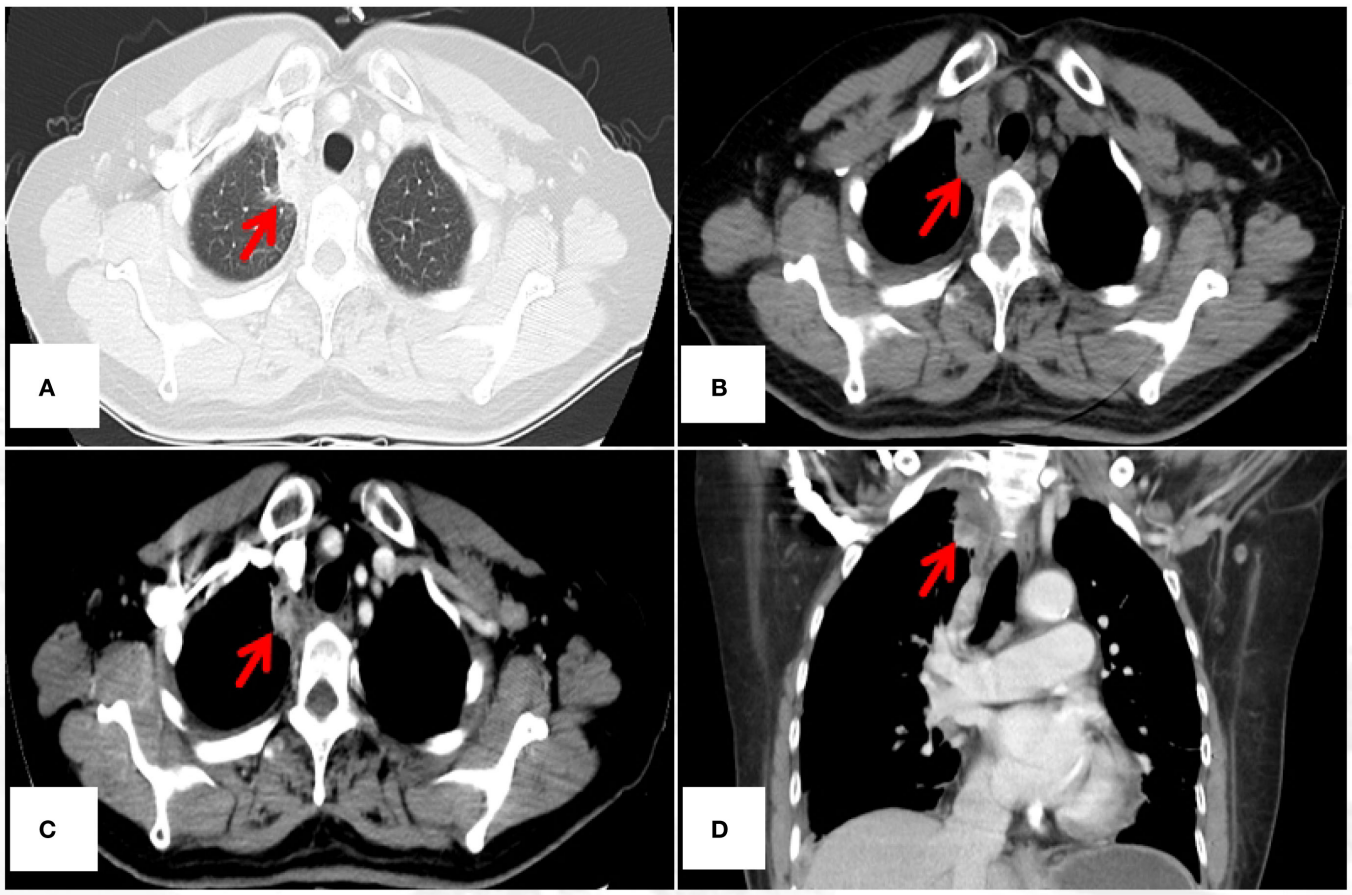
45-year-old female diagnosed with ETAC. Immunocytochemistry shows: Ber - EP4(+), CK7(+), TTF - 1(SPT24)(-), Napsin A(-), Villin(+), CDX - 2(+), GATA3(-), PAX8(-), CR(-). (A) Lesion under lung window (arrow); (B) Lesion under mediastinal window non – contrast (arrow), (C, D) Lesion under mediastinal window contrast - enhanced, showing heterogeneous enhancement(arrow). CT, computed tomography.
